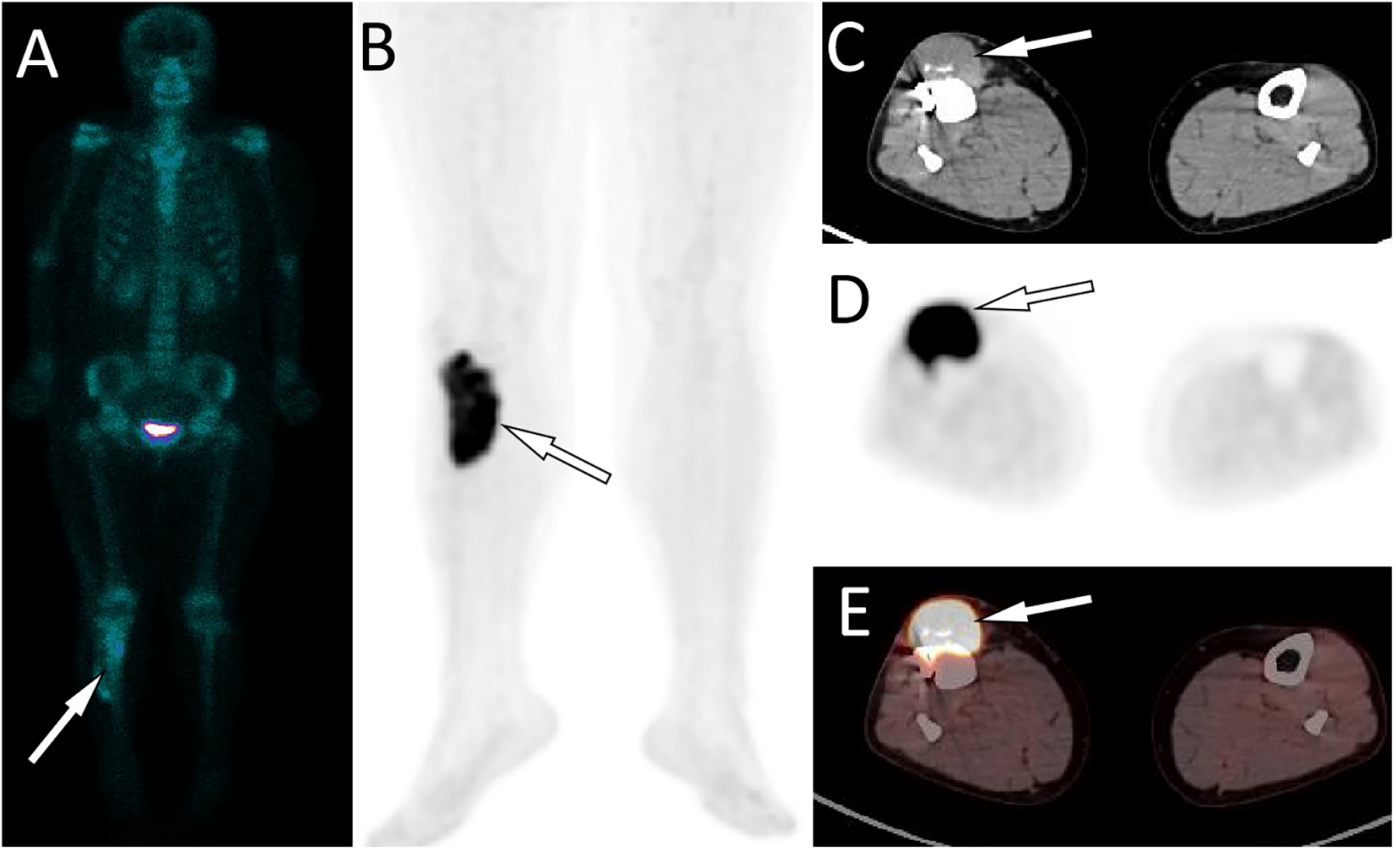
^99m^Tc-MDP SPECT whole body bone imaging showed no significant radioactive concentration except for the right proximal tibia (A, arrow). The PET/CT examination of the patient about 2 years after surgery revealed an increased ^18^F-FDG uptake in the surgical area of the original proximal tibial lesion (B, arrow). The axial CT (C) at the corresponding position reveals a soft - tissue mass shadow of uniform density at the anterior edge of the original proximal tibial surgical area (arrow), measuring approximately 4.0 cm × 1.8 cm × 8.5 cm in size. It is accompanied by a significantly increasded ^18^F - FDG uptake on the axial PET (D, arrow) and PET/CT fusion (E, arrow), with a SUVmax of 14.1.
